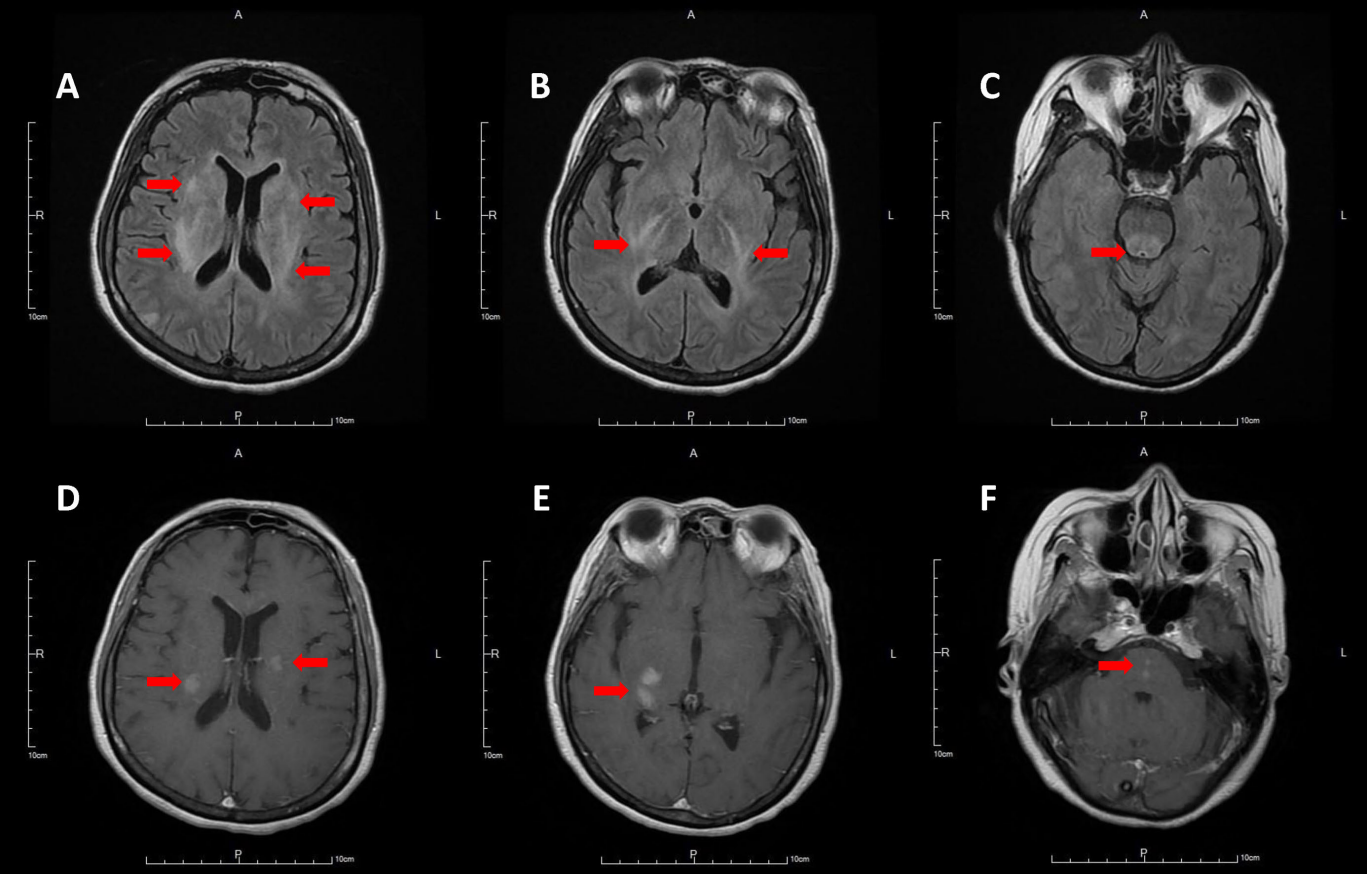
Hyperintensities involving bilateral subcortical white matter and basal ganglia (A), posterior limb of internal capsule (B), brainstem (C) on T2 fluid attenuated inversion recovery brain MRI, with contrast enhancement on post-contrast T1-weighted images (D–F).
